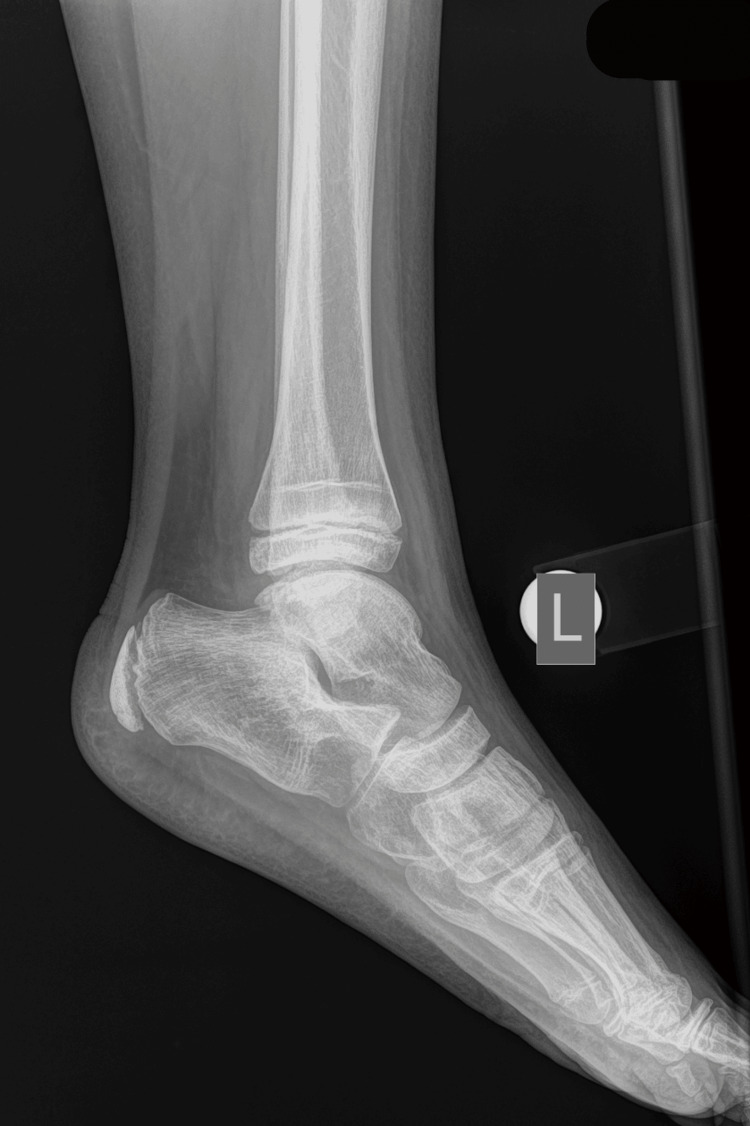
Lateral X-ray of the left injured ankle
